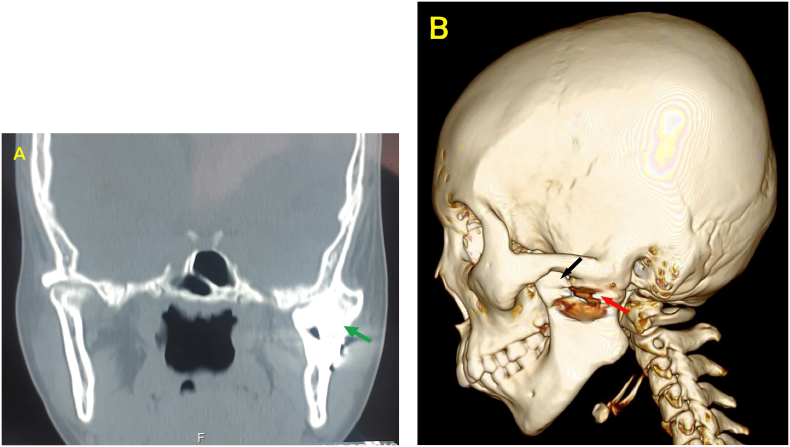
A CT image showed ankylotic bone mass, and there was no space between the glenoidal fossa and the left mandibular ramus (green arrow), B 3D CT image showed reankylosis which was characterized by bone growth between the glenoidal fossa and the left mandibular ramus. The gap has been closed by bone mass that extends covering the left mandibular incisura (red arrow). Hyperplasia left coronoid processus was visible (black arrow). (For interpretation of the references to colour in this figure legend, the reader is referred to the web version of this article.)
